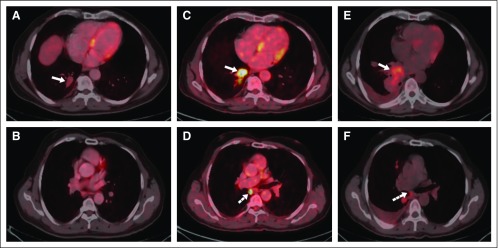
Transaxial fused [^18^F]fluorodeoxyglucose (FDG) positron emission tomography–computed tomography images. (A and B) After 3 years of erlotinib treatment (May 2013), a 2.6-×-1.7-cm FDG-avid nodule (maximum standardized uptake value [SUVmax], 2.7) in the right lower lobe (RLL; arrow), non–FDG-avid subcentimeter subpleural nodules, and non–FDG-avid right pleural thickening were visible. (C and D) After 6 years and 3 months of receiving erlotinib treatment (August 2016), an interval increase in metabolic activity of the RLL nodule (arrow; SUV max, 8.0) and subcarinal lymph node (dashed arrow; SUV max, 9.8) were visible. (E and F) In February 2018, an interval increase in size and metabolic activity of the RLL mass (arrow), with right subcarinal lymph node (dashed arrow) and reappearance of right pleural effusion, were visible.
